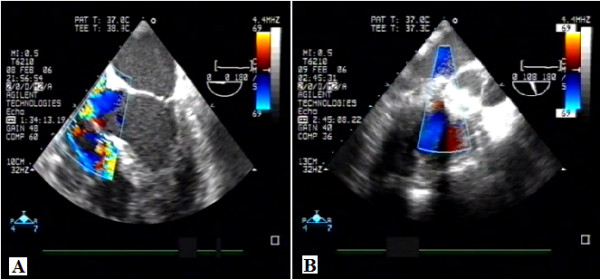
Intraoperative transesophageal echocardiography pre and after device implantation. A. Intraoperative transesophageal echocardiography demonstrating an important left to right shunt and B. Intraoperative transesophageal echocardiography demonstrating non residual left to right shunt after the device implantation.
